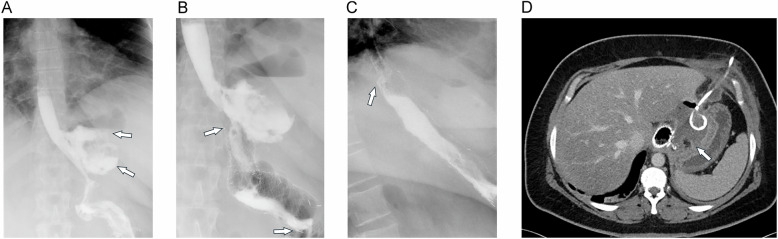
Anastomotic leak with abscess and CT-guided drainage. A Upper GI study shows large contrast extravasation at the anastomosis and a large extraluminal gas collection (arrows). B A stent was placed, which did not seal the leak due to distal migration (arrows indicate upper and lower ends of the stent). C After placement of a second (more proximal) stent (arrow indicates proximal end of new stent), no extravasation is seen. There are still some small residual distant extraluminal gas bubbles. D Due to persistent fever, MDCT was performed, which shows a large abscess (arrow), which was subsequently drained under CT-guidance
